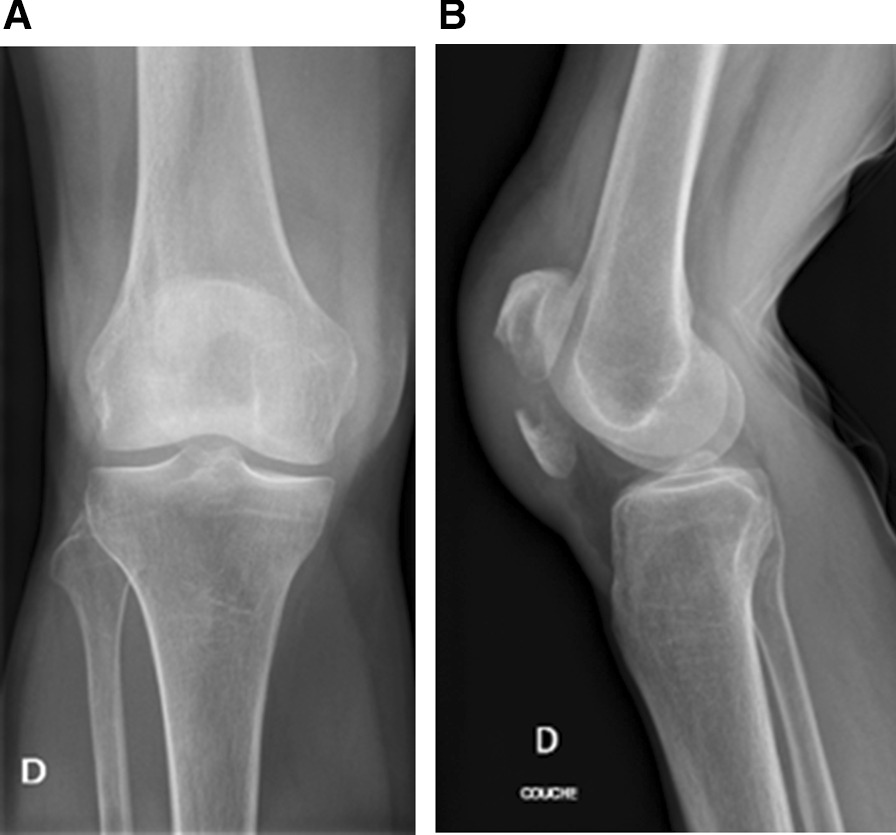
a, b Distal avulsion fracture of the right patella, x-rays before surgery
